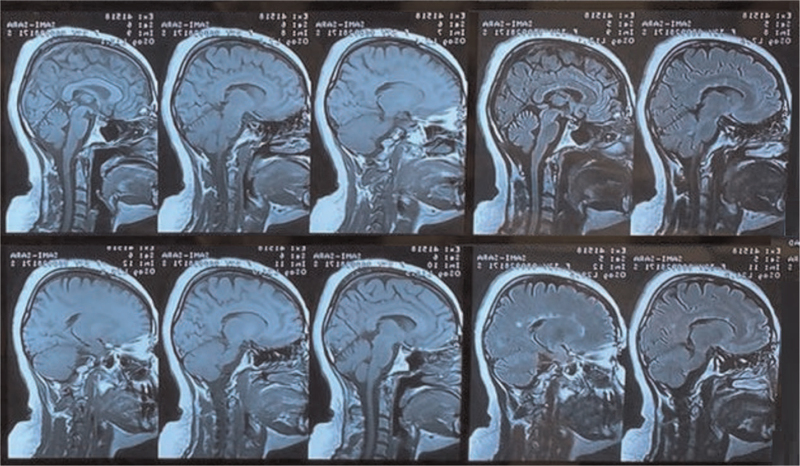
Magnetic resonance imaging demonstrating multiple sagittal section views of a multiple sclerosis patient brain highlighting in black arrows typical multiple white matter plaque lesions in the periventricular region of the brain.
